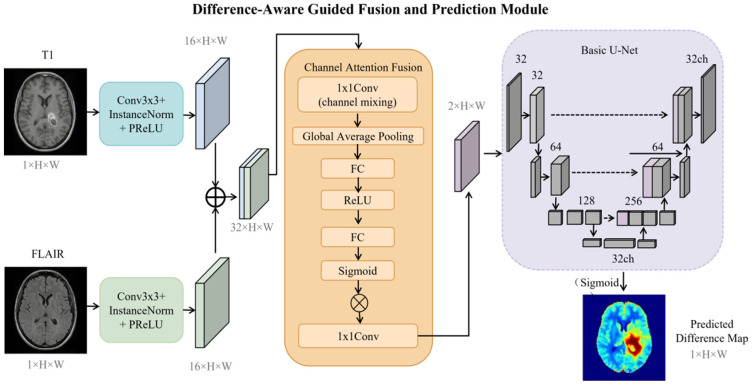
Structure of the Difference-Aware Fusion and Prediction (DAFP) module. T1 and the auxiliary sequence are first processed by two independent convolutional branches for low-level feature extraction. The extracted features are fused through a cross-modal channel attention mechanism and projected into a compact 2-channel bottleneck guidance representation. This representation is then decoded by a Basic U-Net predictor to generate the final single-channel lesion-related discrepancy map used to guide subsequent CE-MRI synthesis.
